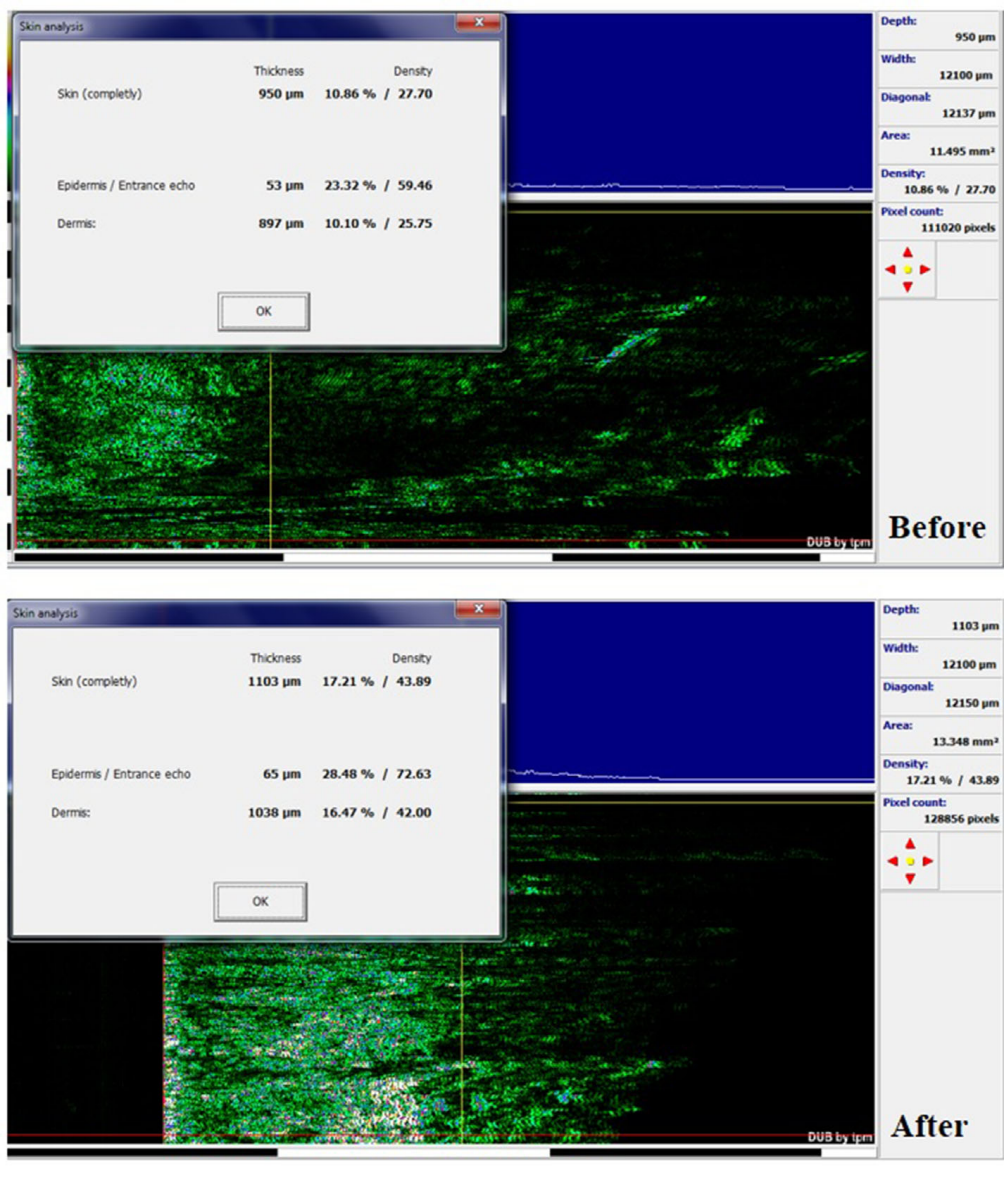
Skin analysis with ultrasonography. The skin density and thickness, before and 6 months after the last SFR treatment session.
